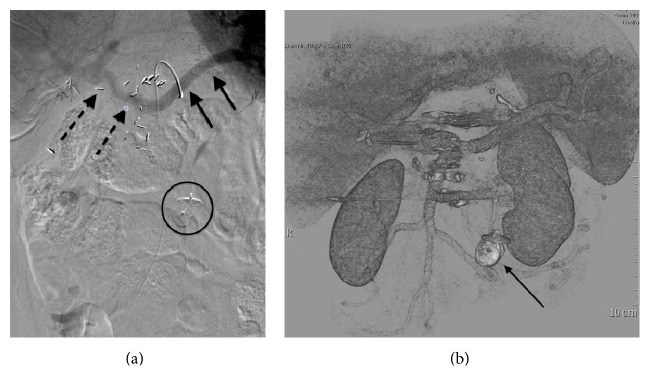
(a) Visceral phase angiography following injection of the splenic artery after occlusion of the portosystemic shunt with Amplatzer vascular plug (circled) demonstrates splenic vein (white arrows) draining antegrade into the portal vein (black arrows). (b) VR CT image shows the position of the Amplatzer plug (arrow) and successful embolization of the shunt (no longer visualized). Portal venous flow is now seen to and within the liver.
